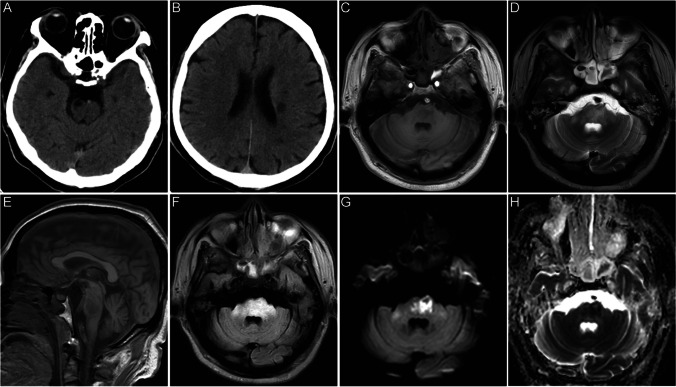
Neuroimaging findings in DQ-induced toxic encephalopathy. A head CT scan showed patchy low-density shadows on the right side of the brainstem (A) and near the left ventricle (B). Multiple patchy long T1 (C) and long T2 (D) signals in the right frontal lobe, bilateral paraventricular, bilateral basal ganglia, brainstem, and right cerebellum. Large low-signal shadows in the T1W sagittal position (F) pons. FLAIR mostly showed high signal (F). DWI (G) brainstem lesions showed significantly high signal, and ADC (H) showed low signal
